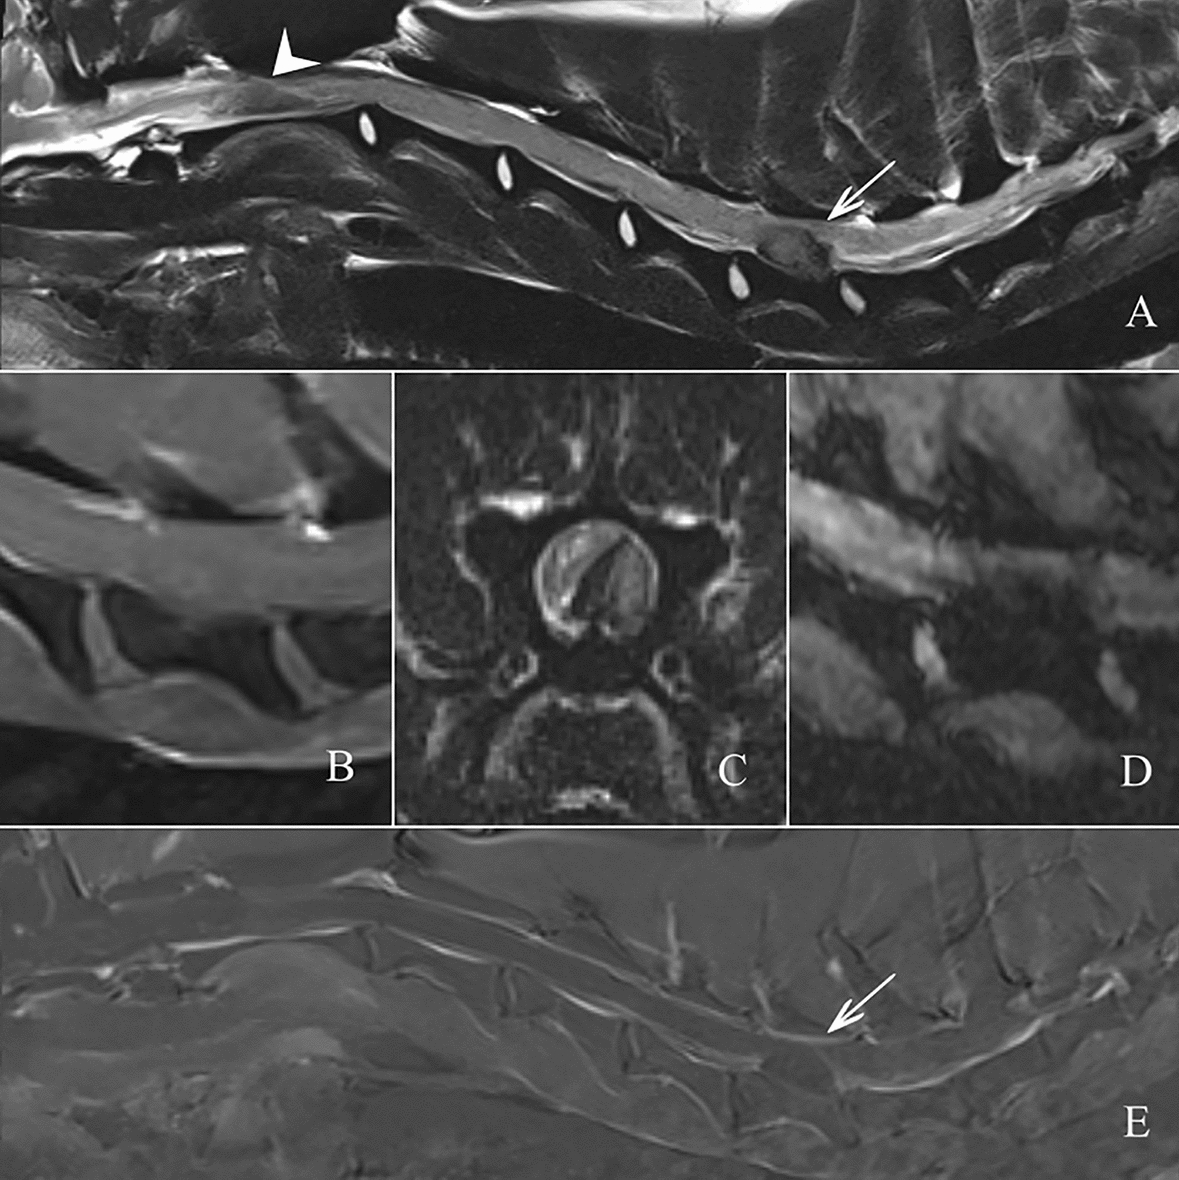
Magnetic resonance imaging of the cervical spine. Extramedullary, extradural, well defined, mildly heterogeneous, oval mass lesion at the level of C6 (arrows). The lesion is hypointense in T2 sagittal (A) and transverse (C), T1 sagittal (B) and mildly hyperintense in STIR. This mass is ventral and right sided, occupying ca. 70% of the vertebral canal with moderate to severe mass effect on the spinal cord that appears displaced towards the left lateral side. The mass shows a mild heterogeneous susceptibility artefact in the sagittal T2 FFE sequence (D) and minimal contrast enhancement in the T1 subtraction sequence (E). At the level of C2 the spinal cord appears increased in volume. In the CISS sequence there is a circumferential heterogeneous subarachnoideal/subdural material encircling the spinal cord with mixed hyper-hypointense areas. This material is moderate hyperintense in T2 (arrowhead, A), STIR and iso- to hypointense in T1. Very mild contrast enhancement is visible (E). Susceptibility artifact is noted
